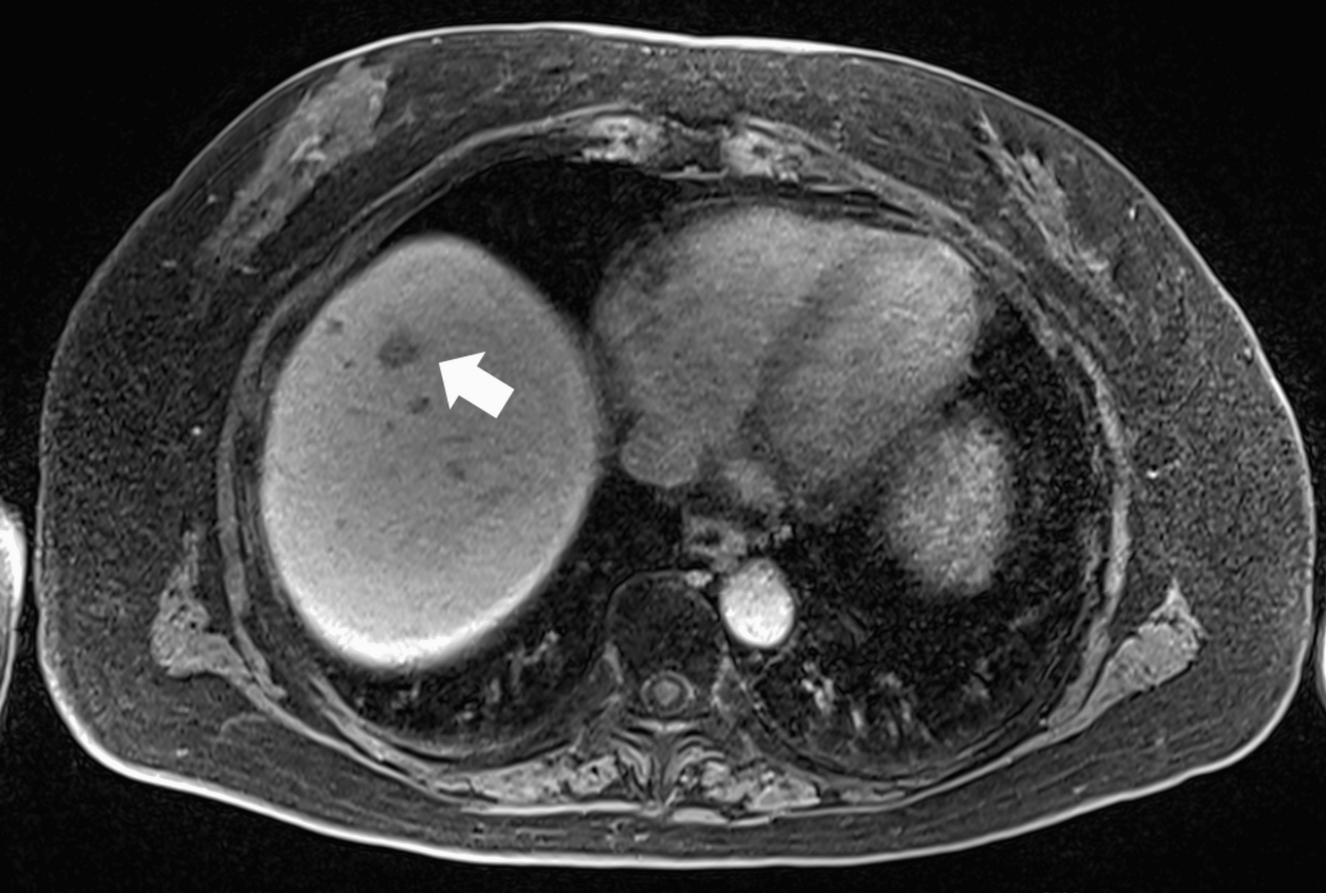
Magnetic resonance imaging T2 hepatobiliary phase of Case 2 showed a small low-intensity mass (arrow) in segment 8 of the liver.
